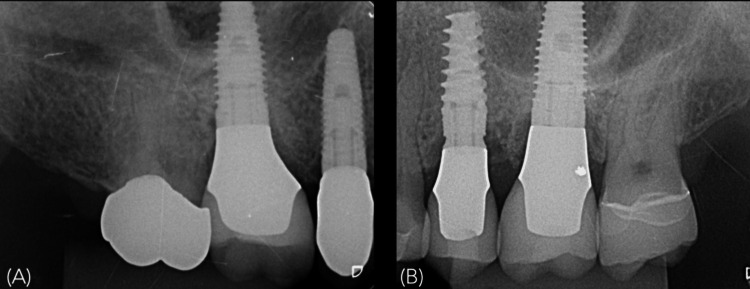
One-year follow-up radiographs Periapical radiograph obtained 12 months after implant placement in (A) Case 1 and (B) Case 2, demonstrating stable peri-implant bone levels.
